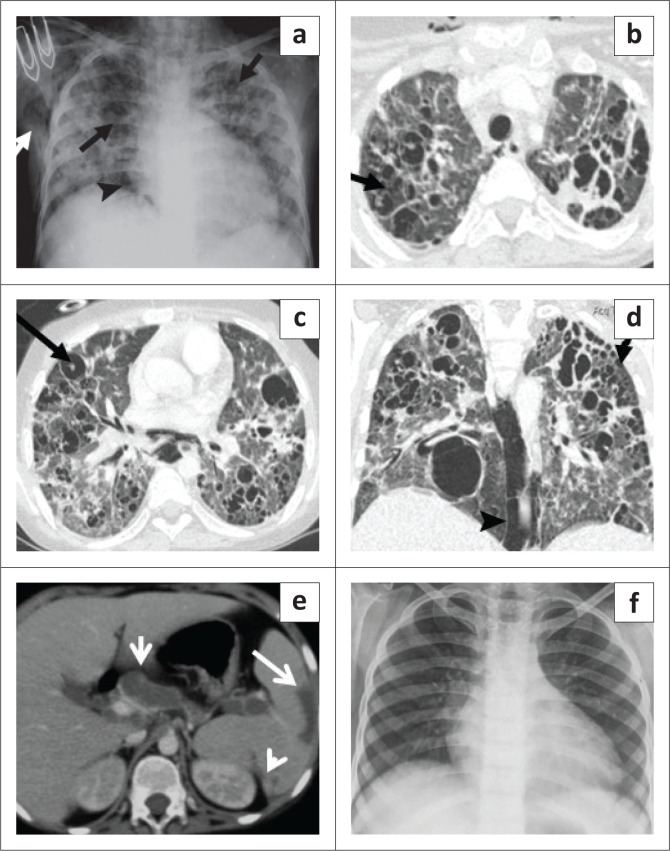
Chest radiography (CXR) (a) shows bilateral patchy lung opacities with multiple cystic lucencies (black arrows), subcutaneous emphysema (white arrow) and pneumomediastinum (arrowhead). Contrast-enhanced CT chest axial (b, c) and coronal (d) images reveal multiple cysts of varying sizes in both lungs (short arrow), pulmonary interstitial emphysema (long arrow) and pneumomediastinum (arrowhead). (e) Axial section through the abdomen shows necrotic periportal lymph nodes (short arrow), splenic infarct (long arrow) and splenic granulomas (arrowhead). (f) Follow-up CXR (after 5 months) appeared normal.
